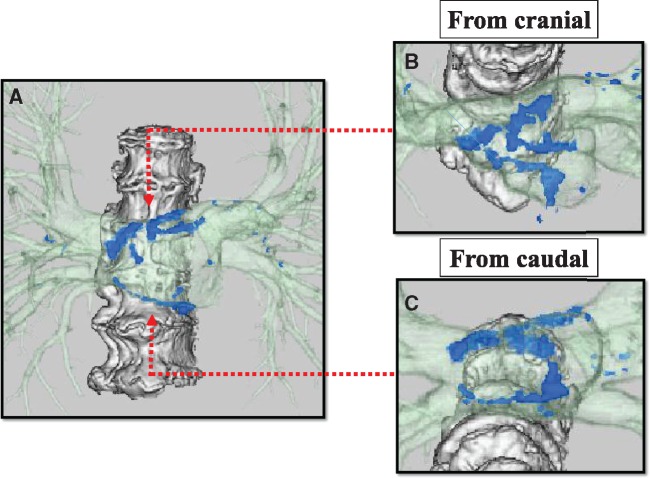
Volume-rendered images from the multidetector row computed tomography are shown (*A*). The structures coloured in light green indicate the left atrium and pulmonary veins, and blue structures represent calcium deposition. Note that the left atrium calcifications were predominantly distributed both at the roof (*B*) and bottom (*C*) of the atrial wall, thereby sandwiching the left atrium.
